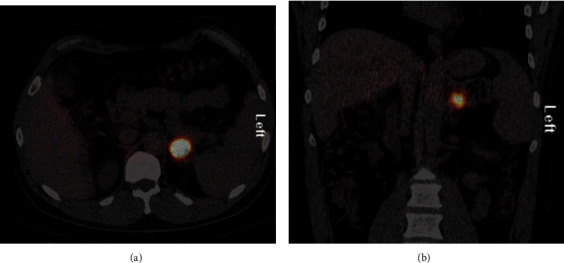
^123^I-metaiodobenzylguanidine (MIBG) scintigraphy scan showed abnormal focalintense radiotracer accumulation in the left adrenal lesion
